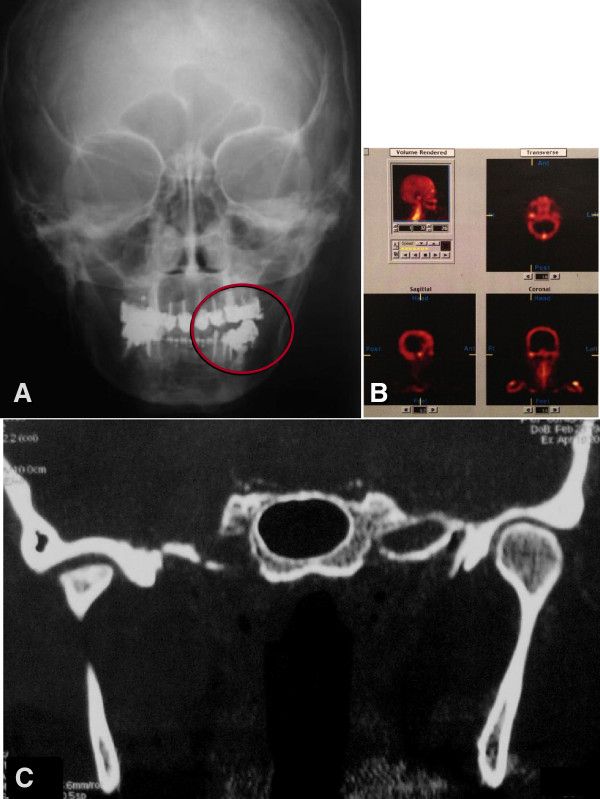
Preoperative evaluation. (A) Frontal initial pre-operative X-ray (frontal teleradiography): An open bite on the left side was present. (B) Bone scintigraphy: High bone metabolic activity was present at the right TMJ. (C) Computed Tomography in coronal sections of the face: Compared to the left side, the right TMJ presented a substantial loss of condyle anatomy associated with areas of bone sclerosis. The asymmetry between the mandibular ramus marks the lateral deviation of the jaw to the right side.
